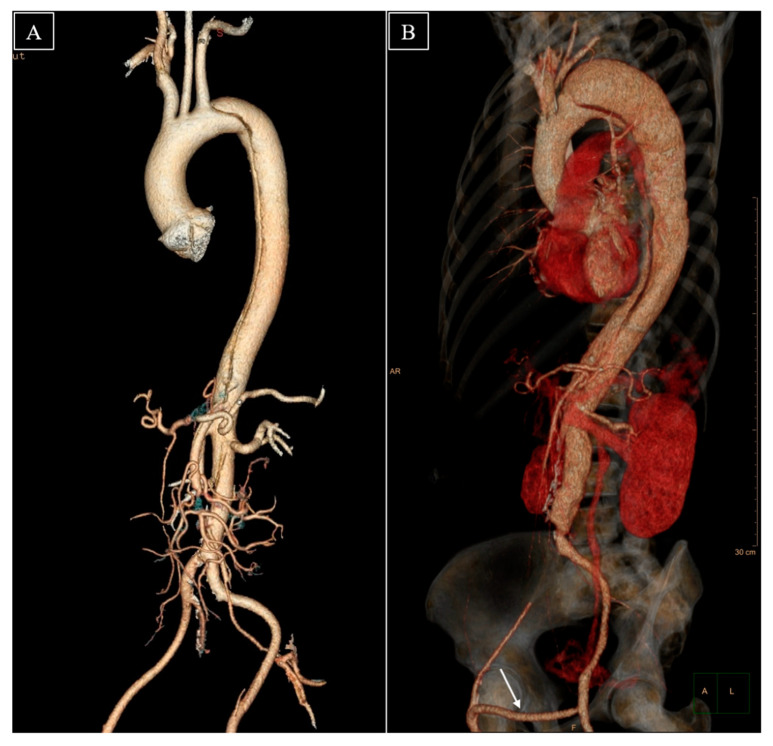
3D computed tomography angiography. (A) The TBAD in 2013. (B) The TBAD in 2020. The white arrow indicates the femoro-femoral crossover bypass graft implanted in 2013.
